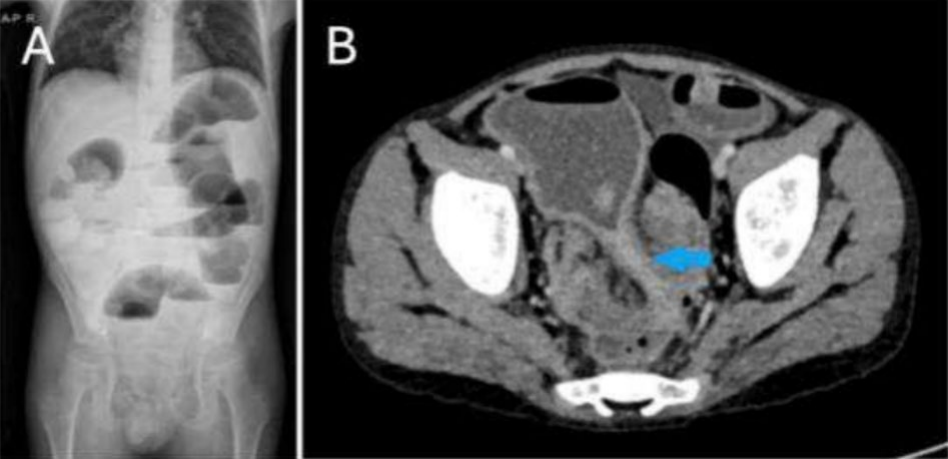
The erect direct abdominal radiograph (A) exhibiting diffuse air-fluid leveling within the colonic loops, indicating ileus. The same patient's axial contrast-enhanced CT scan (B) revealed substantial rectosigmoid wall thickening, resulting in lumen narrowing (arrowed area) and colonic loop dilation proximally in Case 3
